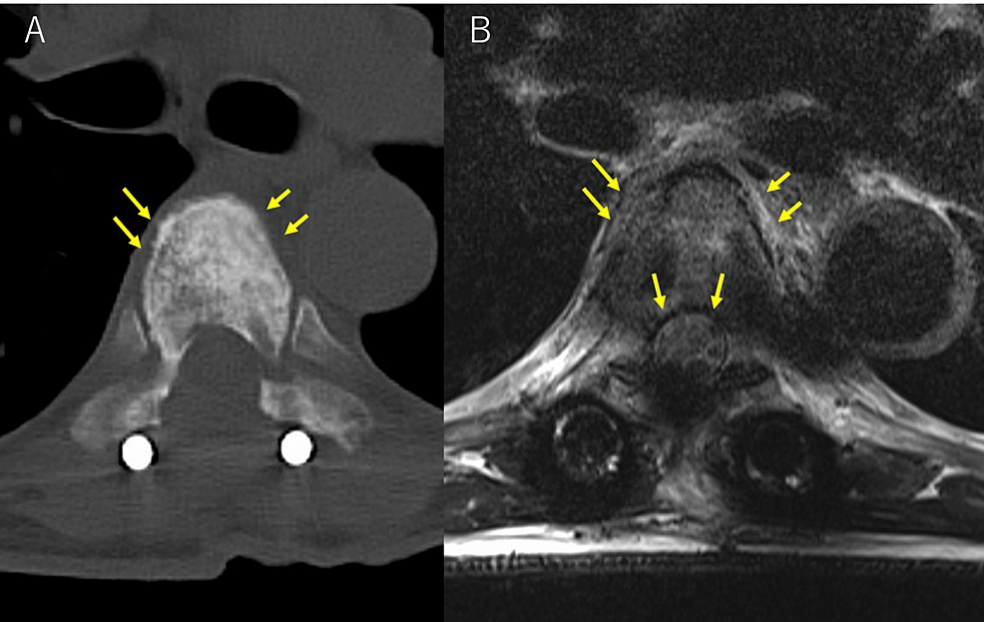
CT and MRI at three months after MTX discontinuation CT (A) and T2-weighted MRI (B) in the axial position confirmed the disappearance of a soft tissue mass extending into the epidural space and paraspinal lesions.
